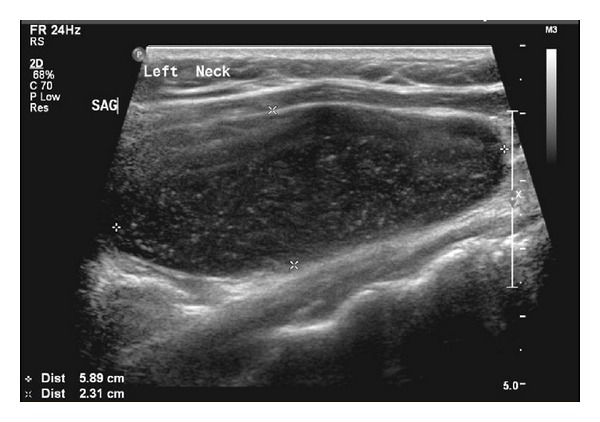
Ultrasound-sagittal view showing the large cystic lesion.
